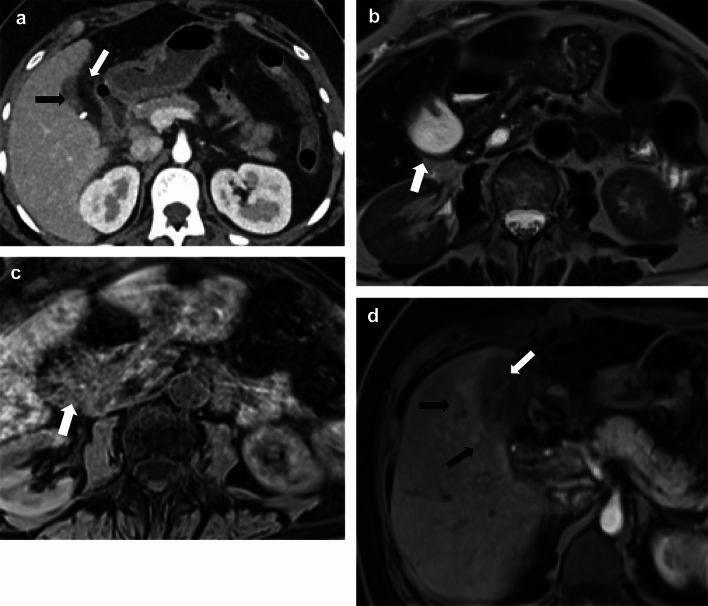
Expected changes post cholecystectomy. a A 27-year-old with abdominal pain post cholecystectomy. An axial CT image showing trace collection (black arrow) and fat stranding in the gallbladder fossa (white arrow), which are expected postsurgical changes. b and c A 75-year-old female with small postsurgical hematoma post-cholecystectomy. b Axial T2 and c Axial precontrast T1W images demonstrate a T2 hyperintense collection that contains intrinsic T1 signal suggestive of blood products (arrow). d A 72-year-old post-recent cholecystectomy. Axial T1W post-contrast image shows reactive hyperemia in the adjacent liver (black arrows). Expected trace collection and fat stranding in the gallbladder fossa (white arrow).
